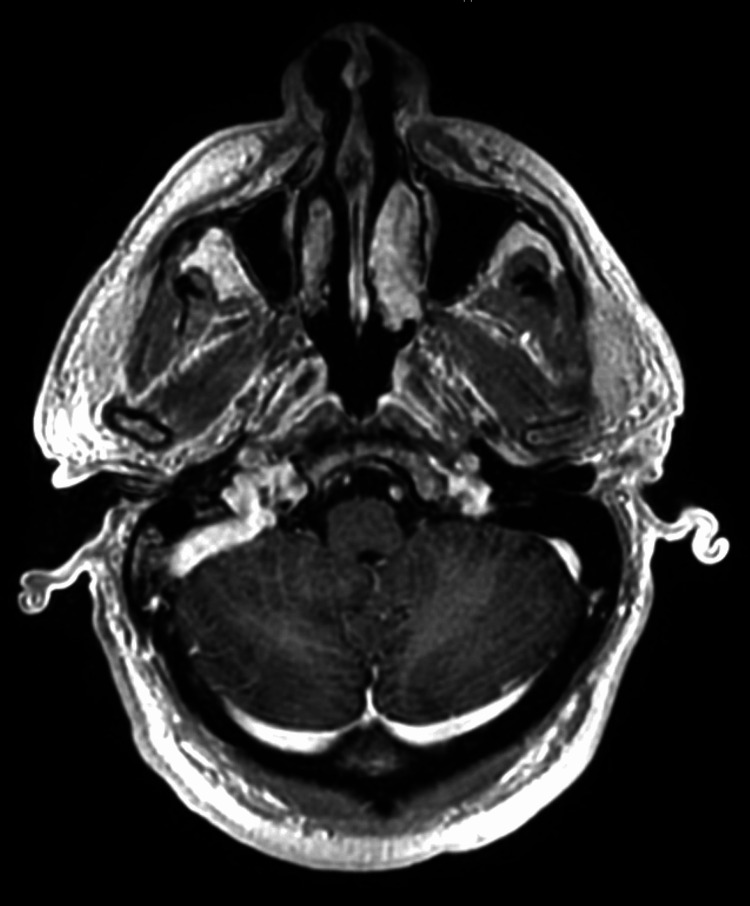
Axial head MRI T1 post-contrast at the level of the cerebellar folia showing no abnormal enhancement or signs of leptomeningeal disease
